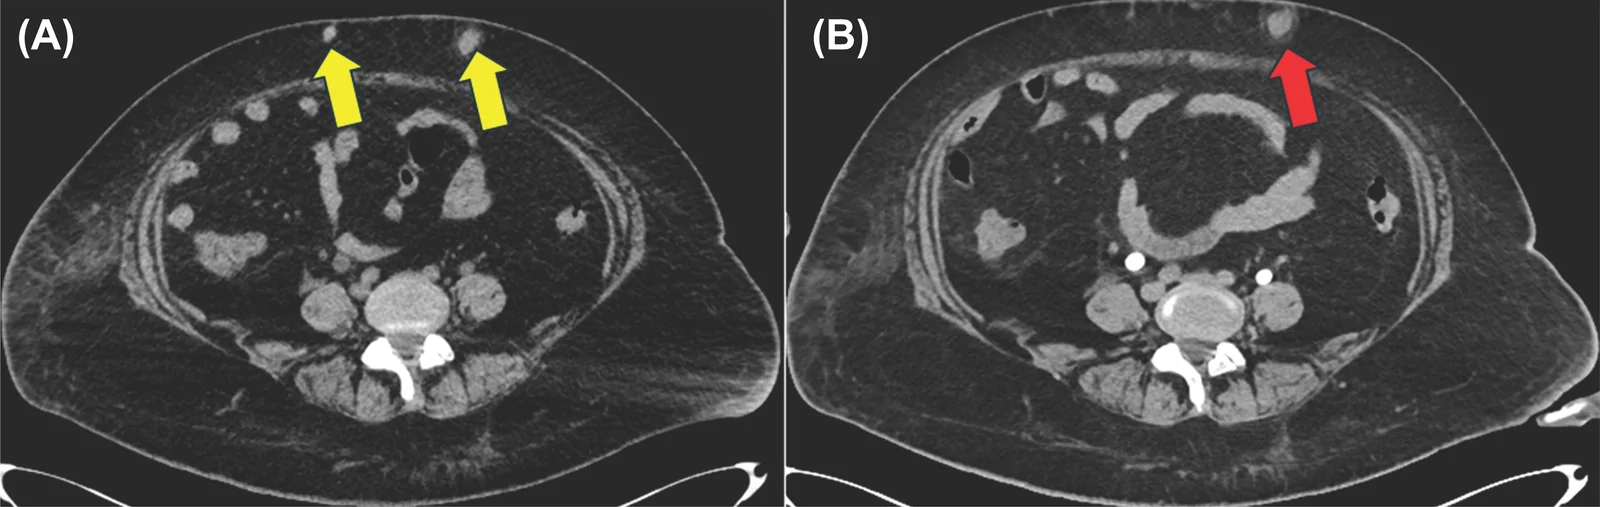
Axial abdominal CT non-contrast (A) and contrast-enhanced images (B). Hyperdense anterior abdominal-wall lesions with hypodense centers (yellow arrows), with some containing air pockets; the largest lesion (17 mm) lacks post-contrast enhancement (red arrow), consistent with granulomatous nodules. Abbreviation: CT, computed tomography.
